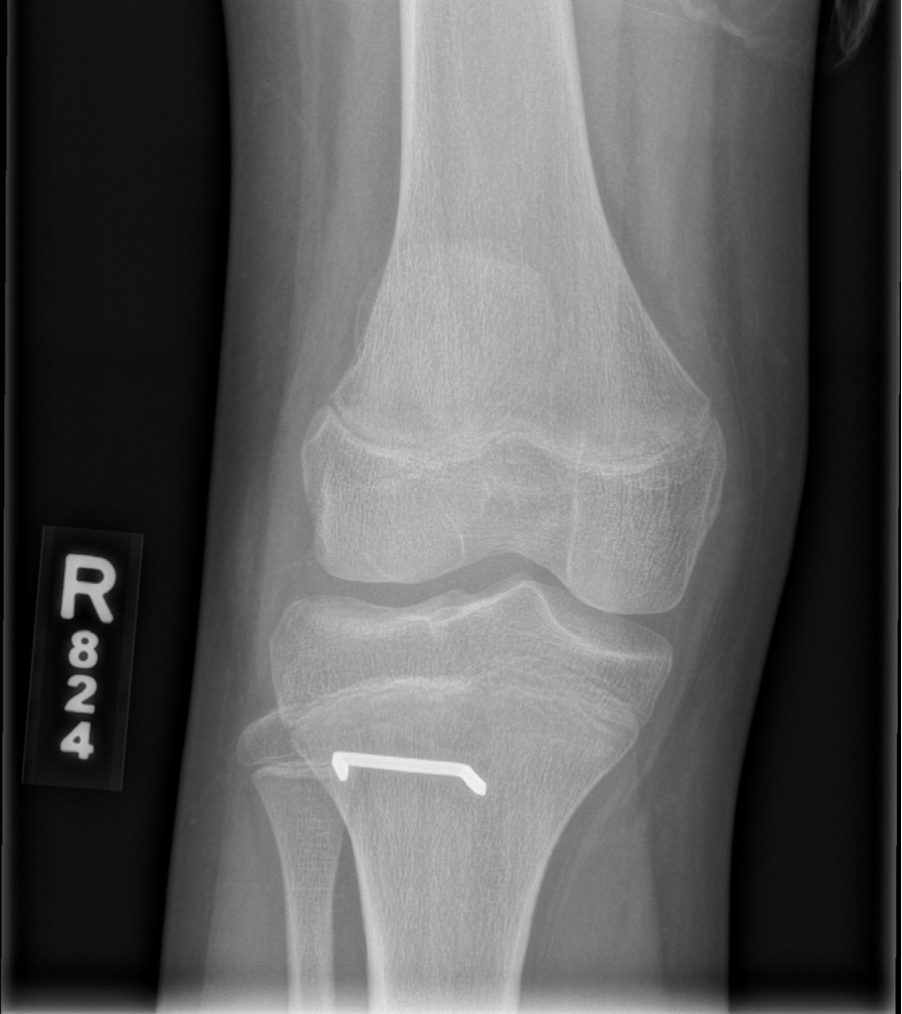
Anteroposterior postoperative radiographs of the patient described in Case 1. Surgical repair including staple fixation of the tibial tubercle avulsion fracture is evident.
